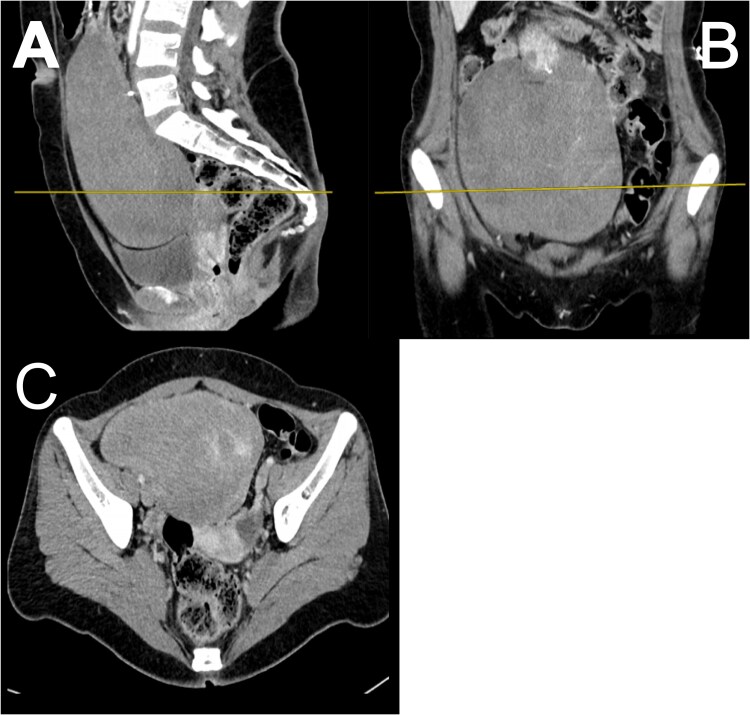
Sagittal (A), coronal (B) and axial (C) CT abdomen and pelvis showing the large mass and likely point of origin from the anteroinferior aspect of the gastric remnant, no evidence of metastasis.
